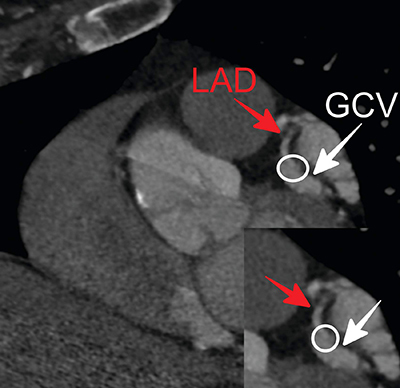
A curved planar reformatted coronary CT angiogram and magnified image show the fistulous connection (white rounds) between the left anterior descending coronary artery (red arrows) and the great cardiac vein (white arrows).
